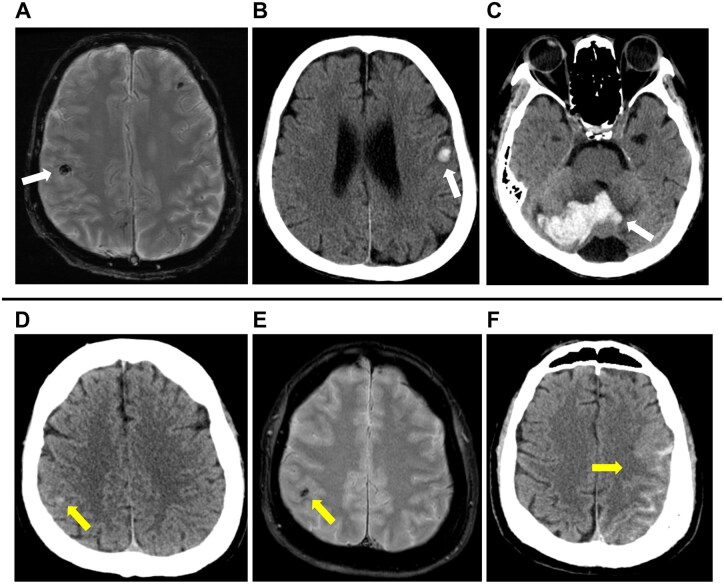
(A) Preoperative Magnetic Resonance Imaging of a Patient with a Small Haemorrhage. Computed Tomography (CT) Showed no Bleeding, but T2* MRI Sequence Revealed a Low Signal Area Measuring ≥10 mm. (B, C) CT on postoperative day 9 revealed cerebellar haemorrhage, subcortical bleeding in the left frontal lobe, and subarachnoid haemorrhage (SAH). (D, E) Preoperative CT and MRI of another patient with small haemorrhage showing bleeding with a diameter of 5 mm. (F) CT taken immediately after surgery revealed SAH
